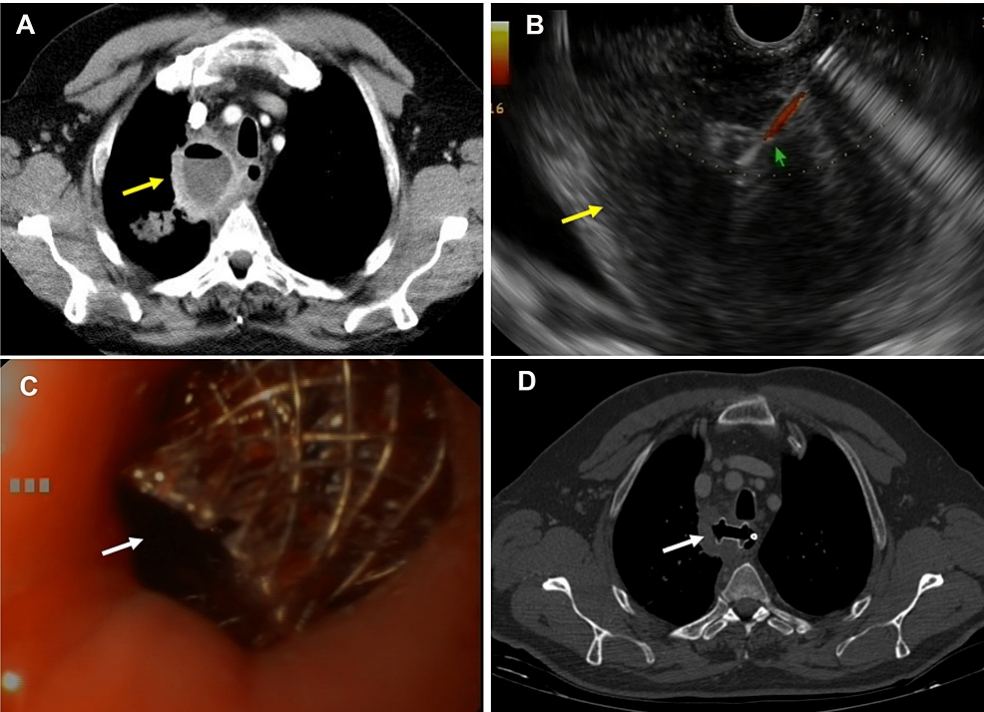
Transmural drainage of mediastinal collection A: Computed tomography scan image of an infected mediastinal collection (note the air-fluid level, yellow arrow). B: Image of endosonography-guided transmural drainage of a mediastinal abscess (yellow arrow); EUS-guided aspiration using a 19 G needle (green arrow) was performed to obtain a sample. C: Endoscopic view of the transesophageal drainage of a mediastinal lesion by placing a lumen-apposing stent (white arrow). D: Computed tomography image of a lumen-apposing stent placed for mediastinal collection of transesophageal drainage (white arrow).
